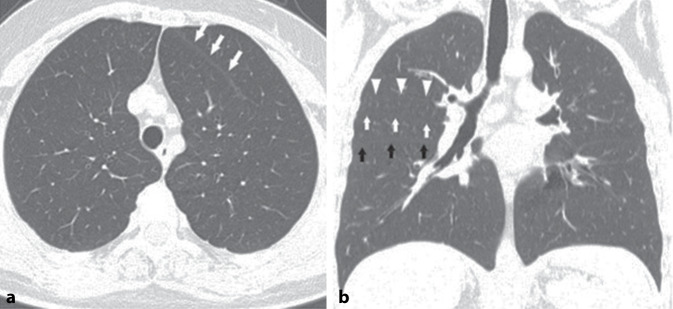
a Accessory horizontal fissure on the left (white arrows indicate the fissure; transverse CT image). b Accessory fissure between the anterior and posterior segments of the upper lobe on the right (white arrows show the horizontal fissure, black arrows show the oblique fissure, white arrowheads indicate the accessory fissure; coronal CT image)
